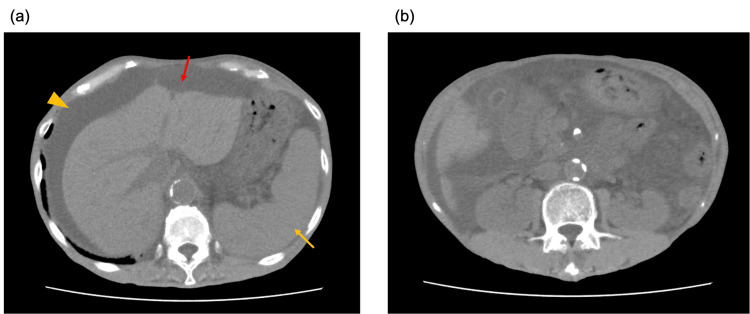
Abdominal computed tomography (a) Deformed liver (suggestive of chronic liver damage; the red arrow), ascites (the yellow arrowhead), and splenomegaly (the yellow arrow). (b) No obvious renal atrophy is observed.
